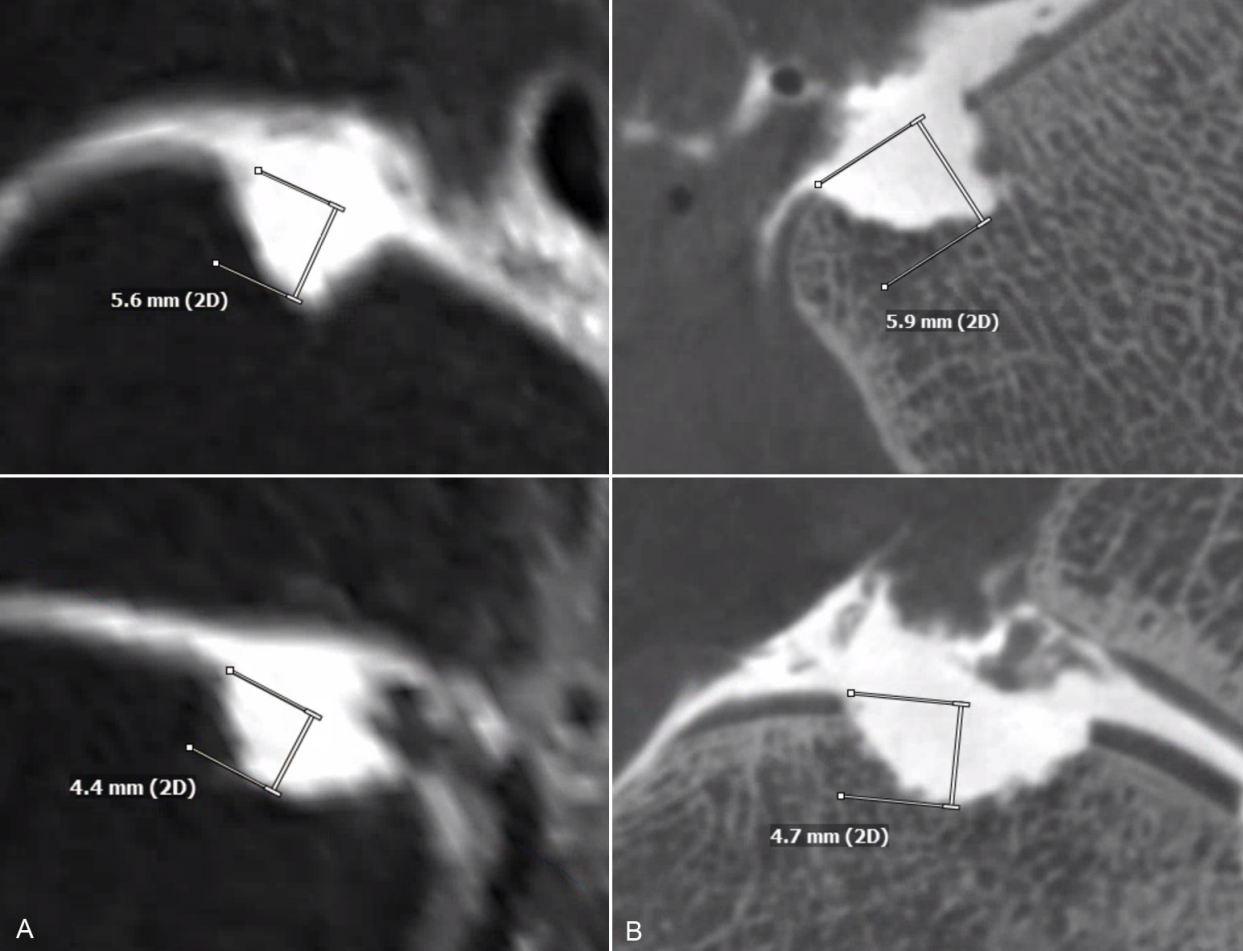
3D MR- and flat panel CT-arthrography measurements. Multiplane reconstruction of one single defect in the isotropic 3D space MR-sequence (A) with the corresponding planes in flat panel computed tomography arthrography (B) with marked depth measurements. The margins of the artificially created defect are clearly defined against the intra-articular contrast agent and the defect dimensions can be measured.
